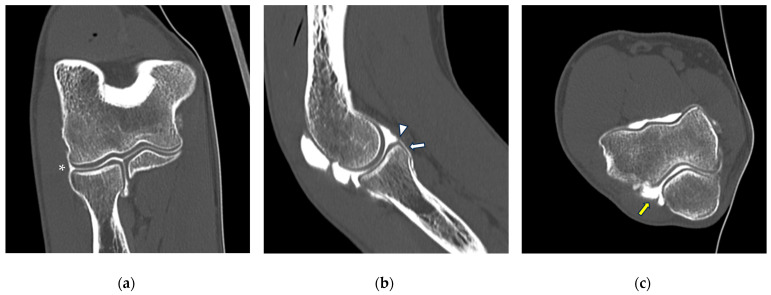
CT arthrography of the elbow—normal anatomy. (a) Coronal image shows a regular chondral lining of the radio-humeral, ulno-humeral, and radio-ulnar joints, with no evidence of focal chondral lesions. The articular capsule is not pathologically distended and there is only a physiological amount of contrast in the lateral radio-humeral recess (asterisk), indicating the presence of an intact radial collateral ligament; (b) sagittal image shows an undisplaced annular ligament (white arrowhead), as well as a regular chondral lining of the radial head side and a physiological sliver of contrast in the annular recess (white arrow); (c) axial image shows a physiological amount of fluid in the posterior recess of the elbow (yellow arrow), with no evidence of loose bodies, as well as regular chondral lining of the humerus and olecranon.
